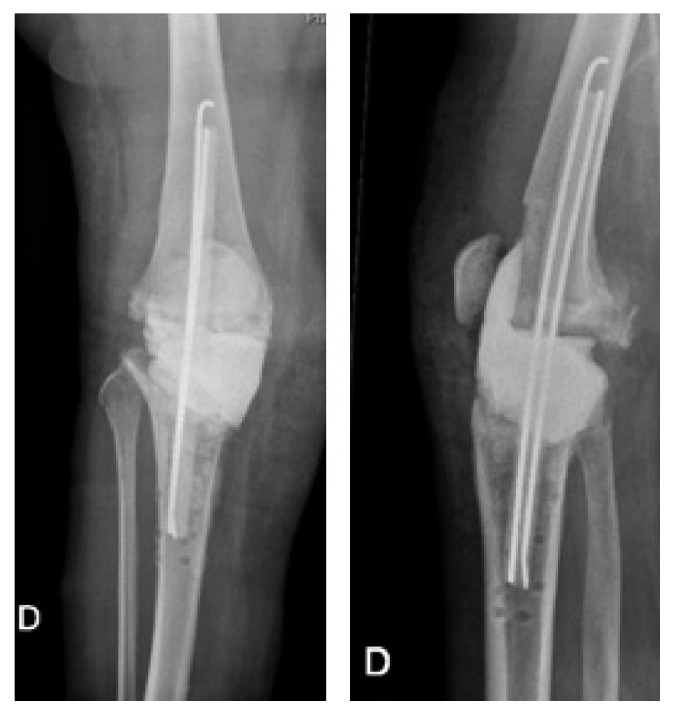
September 2013, X-rays showing postarticular resection and implantation of cement spacer.
